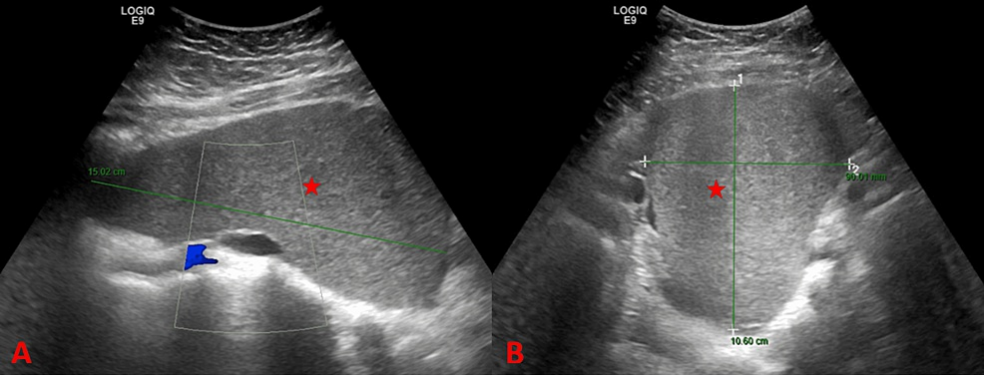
Ultrasound of the patient at the time of admission. A and B: Ultrasound of the lower abdomen and pelvis, showing a large mass occupying the lower abdomen and pelvis with echogenicity similar to spleen (red star).
